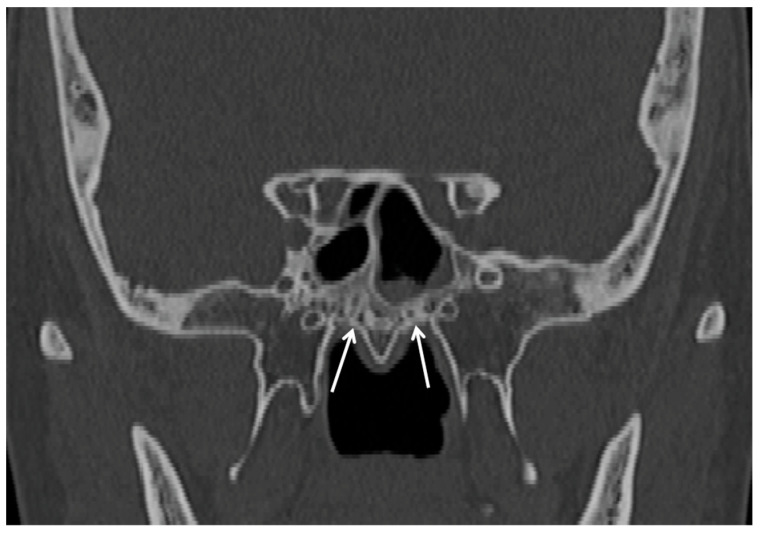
Coronal CT scan of an individual with bilateral palatovaginal canal (white arrows).
